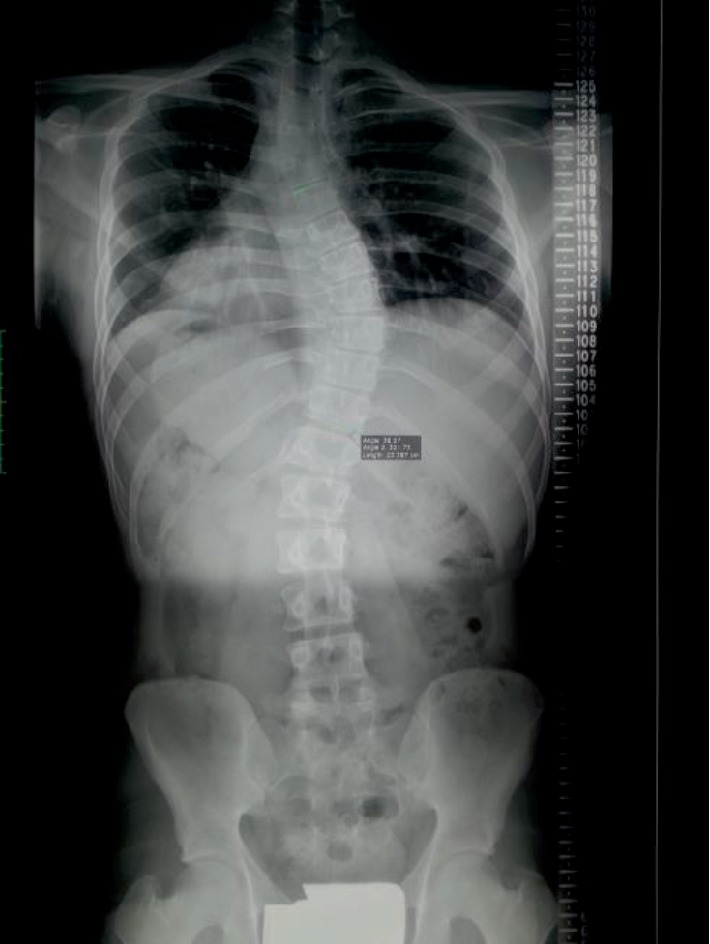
Standing P-A radiograph of 13-year-old girl with right thoracic idiopathic scoliosis. Cobb angle 38°. The girl was referred to consider treatment using corrective bracing.
